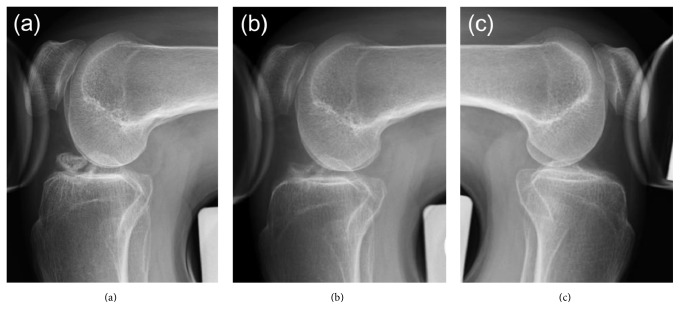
Stress radiographs with anterior drawer tests showing no difference between the (a, b) right and (c) left knees at (a, c) initial examination at our hospital and (b) 2 years after surgery.
